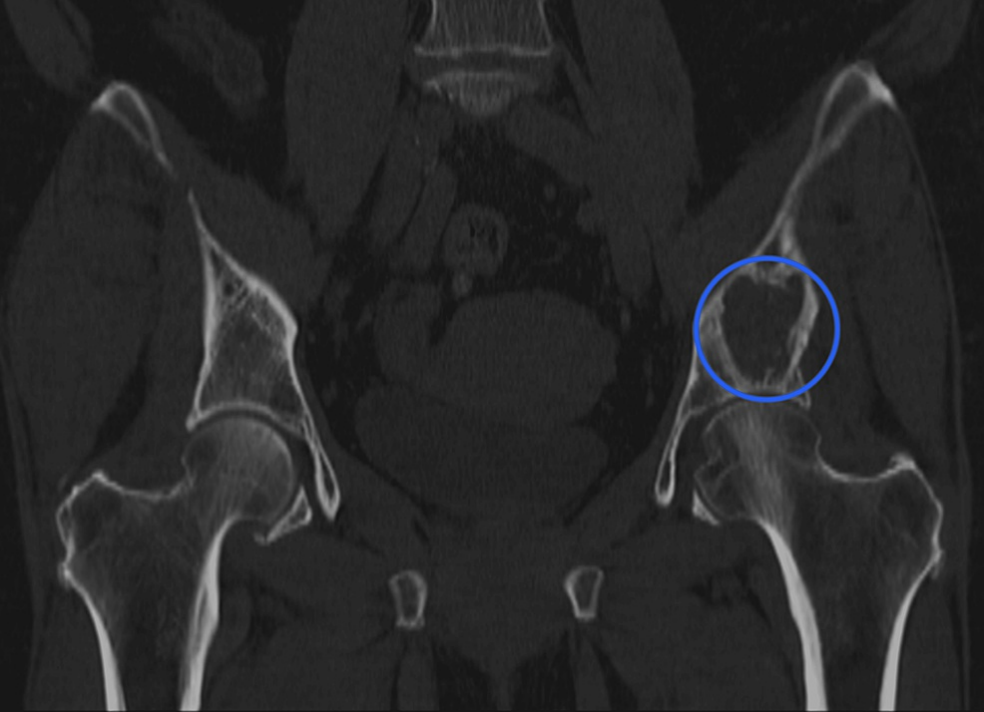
Coronal non-contrast computed tomography image of the pelvis demonstrating a lytic lesion (blue circle) within the left ilium acetabulum.
